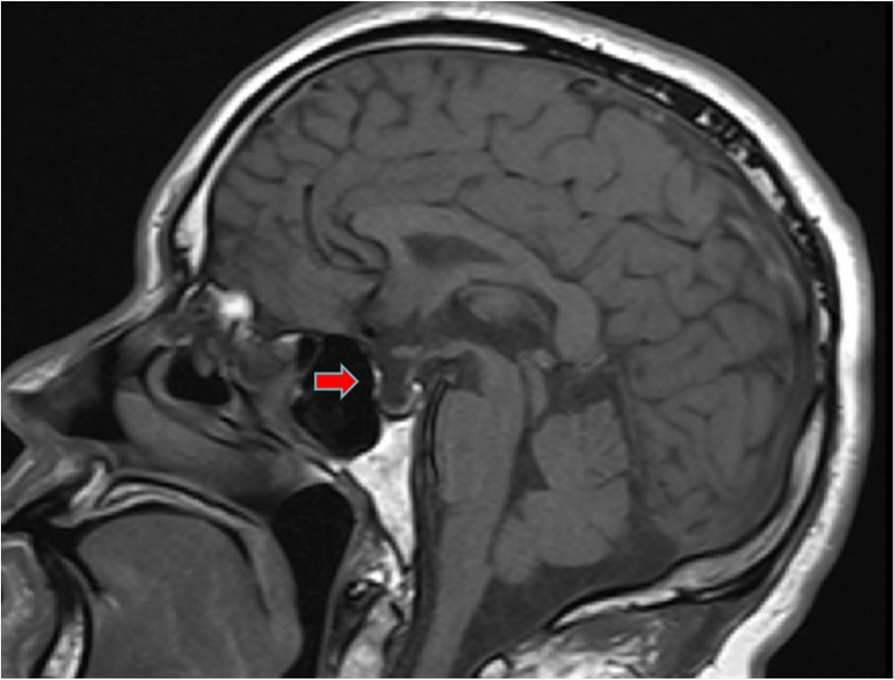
An empty sella on enhanced magnetic resonance imaging of the pituitary
